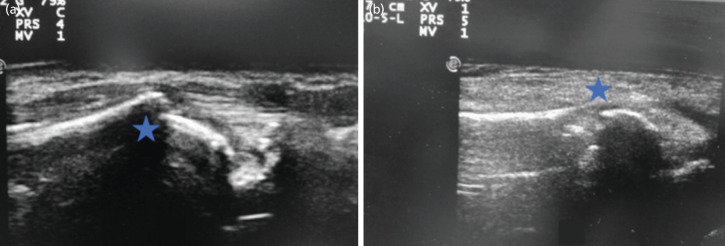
(a, b) Ultrasound before and after reduction in a case of distal radius extraarticular fracture.
